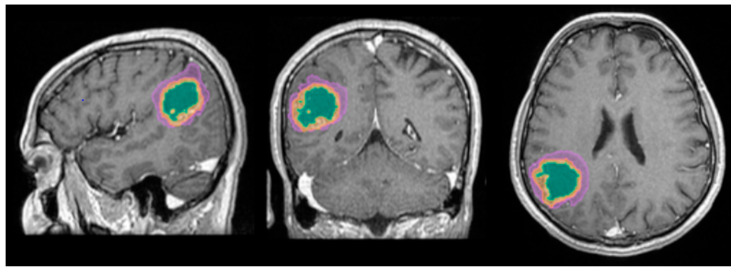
Mdbrain processing of MRI brain acquisition (DICOM) (Scale 1:20). T1W CE in sagittal, coronal and axial planes, respectively. View of the three regions that were calculated separately for every patient: green—necrosis, orange—enhancing part of the tumor and purple—perilesional edema (approval was obtained from the Ethics Committee of the University of Medicine and Pharmacy “Grigore T. Popa” Iasi).
